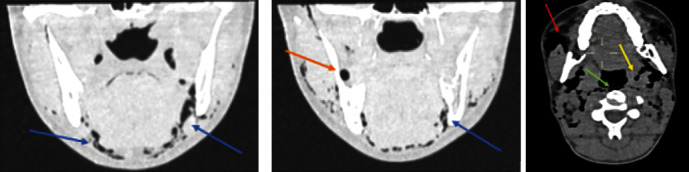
Cervicofacial computed tomography scan of the frontal and axial sections. Air is present in the extraction site of 48 (orange arrow) and in the submandibular (blue arrows), retropharyngeal (green arrow), parapharyngeal (yellow arrow), and masticatory spaces (red arrow).
